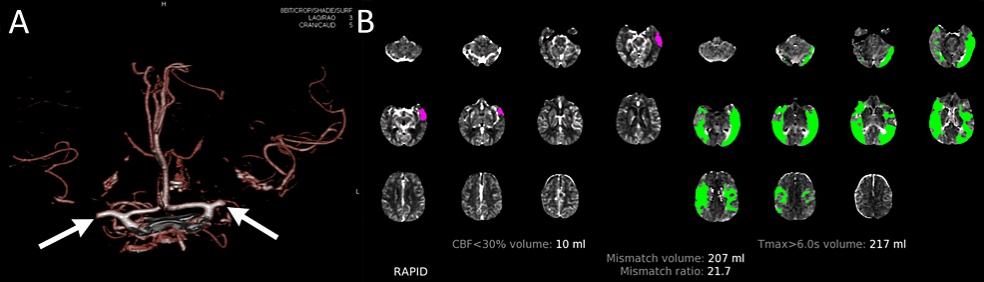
CT angiography and perfusion imaging (A) CT angiography demonstrated proximal middle cerebral artery (MCA) occlusions (arrows) with distal MCA-territory collaterals. (B) CT perfusion demonstrated large area of penumbra (green) with small anterior left temporal core infarct volume (pink).
